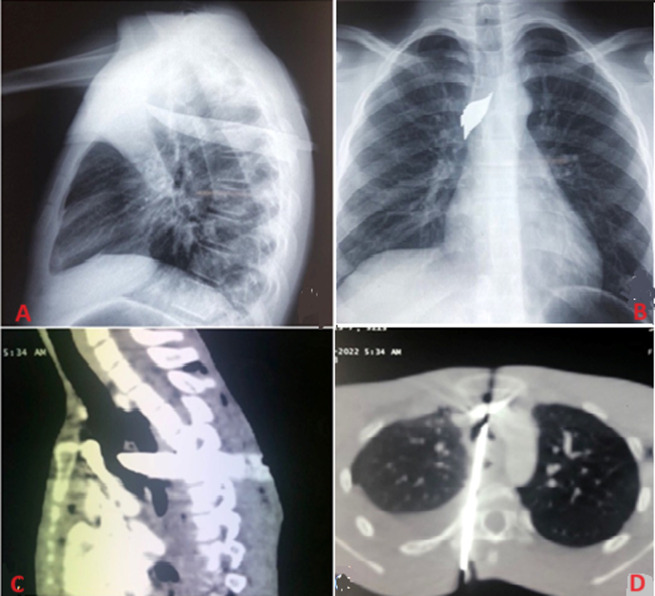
imagerie montrant le couteau dans le thorax; A, B) radiographie de thorax de face et de profil montrant le couteau en intra-thoracique; C, D) scanner thoracique montrant le couteau traversant l´axe aéro-digestif, un hémo-pneumothorax minime à droite et un emphysème sous-cutané
